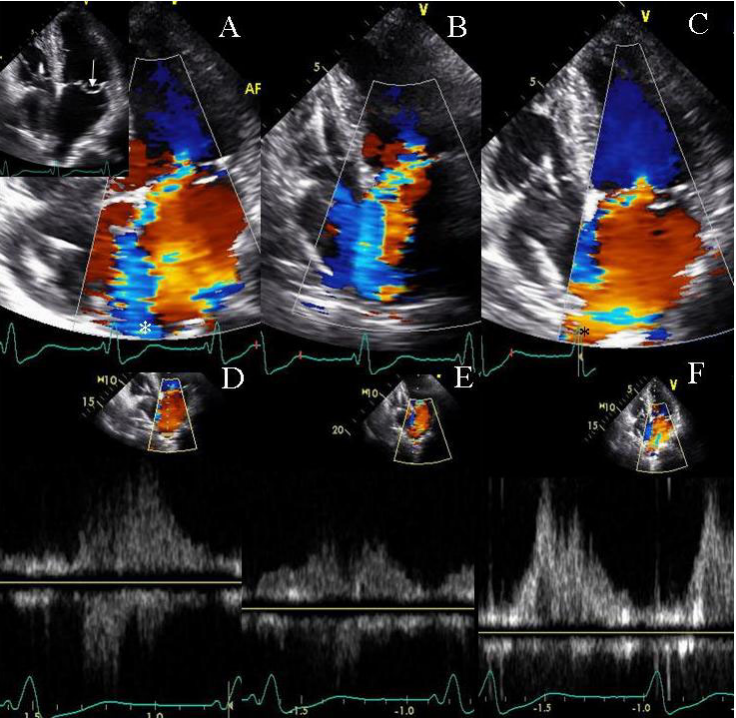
Panels A-C show color Doppler images of mitral regurgitation in the apical 4 chamber view and D-F show right upper pulmonary vein pulsed wave Doppler, at baseline with an AV of 180 ms and LV pre-excitation of 20 ms (A and D), during an AV delay of 220 ms and VV delay of 0 ms (B and E) and during an AV delay of 210 ms and RV pre-excitation of 20 ms. Inset in panel A shows prolapse of the middle segment of posterior leaflet (white arrow) causing malcoaptation between anterior and posterior leaflets and an eccentric severe anterior mitral regurgitation jet entering the right superior pulmonary vein (white asterisk, A) and panel D shows systolic pulmonary vein flow reversal. Prolonging the AV delay to 220 ms no longer causes MR jet to enter the pulmonary vein (B) which in turn produces a somewhat blunted but upright pulmonary vein S wave (E). Right ventricular pre-excitation causes further reduction in mitral regurgitation severity with a dominant systolic flow entering the left atrium (orange red color marked with a black asterisk in C) and a systolic dominant pulmonary vein S wave in F.
